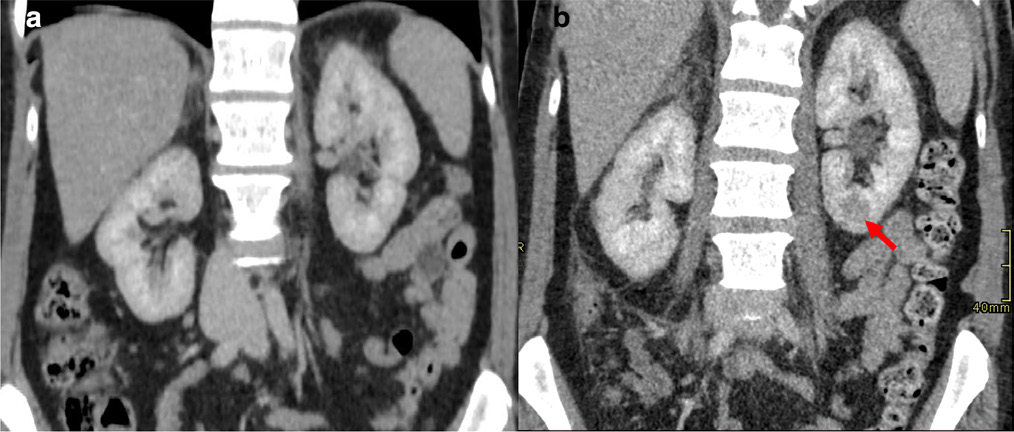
(A) Initial coronal CT of the abdomen on day of admission showing normal enhancement of the renal parenchyma. (B) Coronal CT of the abdomen showing a focus of renal cortical hypoenhancement (arrow) Day 5 post-admission.
